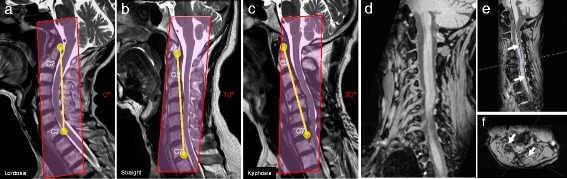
Images showing the cutting angle for MR imaging and the mapping of the root on the workstation. Images show a spine with cervical lordosis (Panel a), a straight spine (Panel b), and a spine with cervical kyphosis (Panel c). The cervical root and ganglion are clearly visible on the double oblique view of the cervical spine (d). The first oblique plane on the sagittal view (Panel e, arrow), and the second oblique plane on the axial view through the right foramen (Panel f, arrow).
